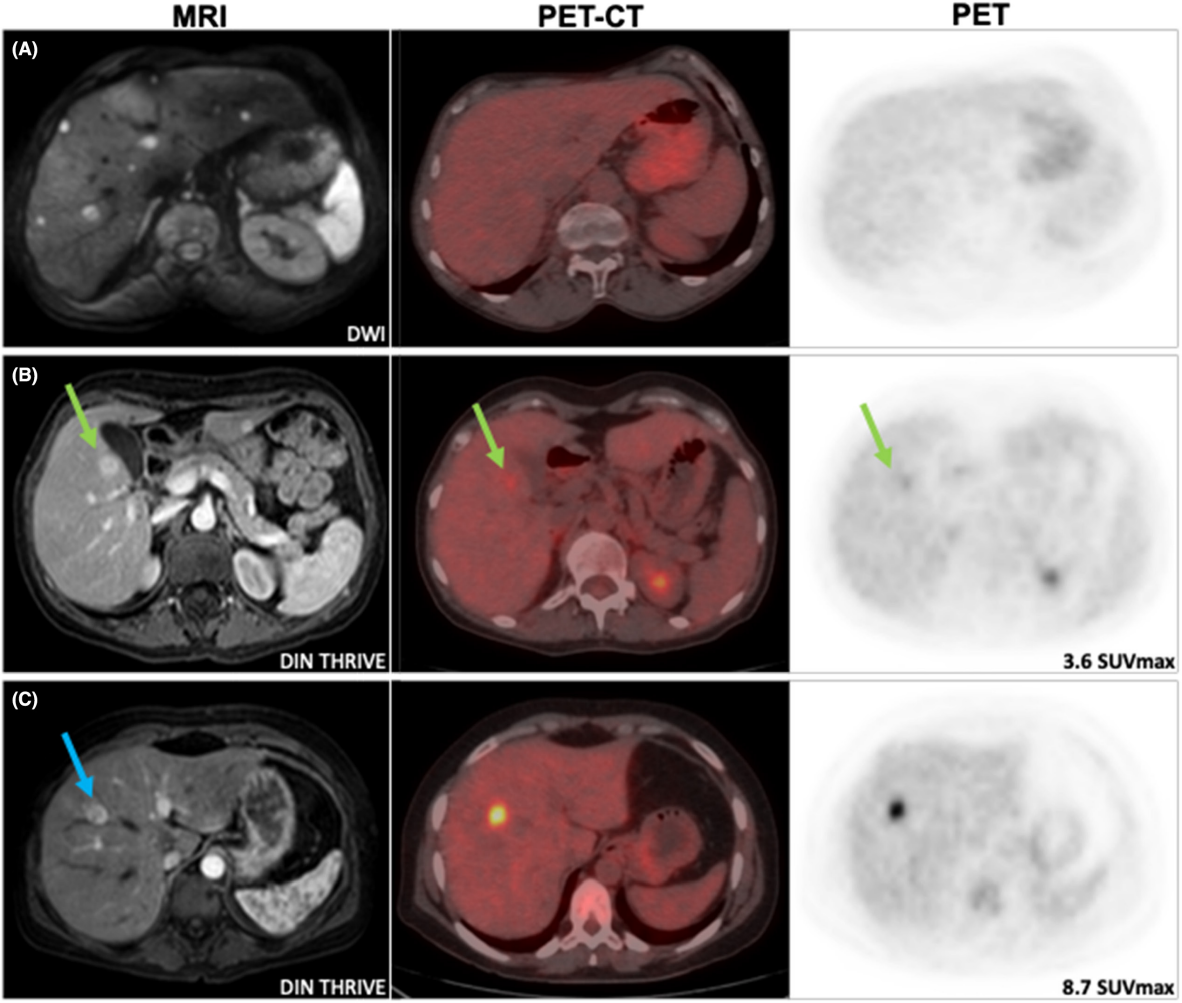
Different degree of FDG uptake in lesions of 15 mm. Lesions are clearly visible in conventional morphological imaging MRI though they can show no increase in FDG uptake with the same activity as healthy liver (A), mild hypermetabolism (green arrow; B), or more significant hypermetabolism (blue arrow; C). All three lesions are depicted as hyperintense in T2 and STIR with a restricted diffusion pattern, and hipervascular after gadolinium I.V. administration in arterial phase by MRI. Lesions are hypointense in T1, with the exception of lesion C that shows central hyperintensity in T1 suggesting melanin deposits in the tumor. CT, computerized tomography; DIN THRIVE, T1W high‐resolution isotropic volume examination; DWI, diffusion weighted imaging; MRI, magnetic resonance imaging; PET, positron emission tomography.
